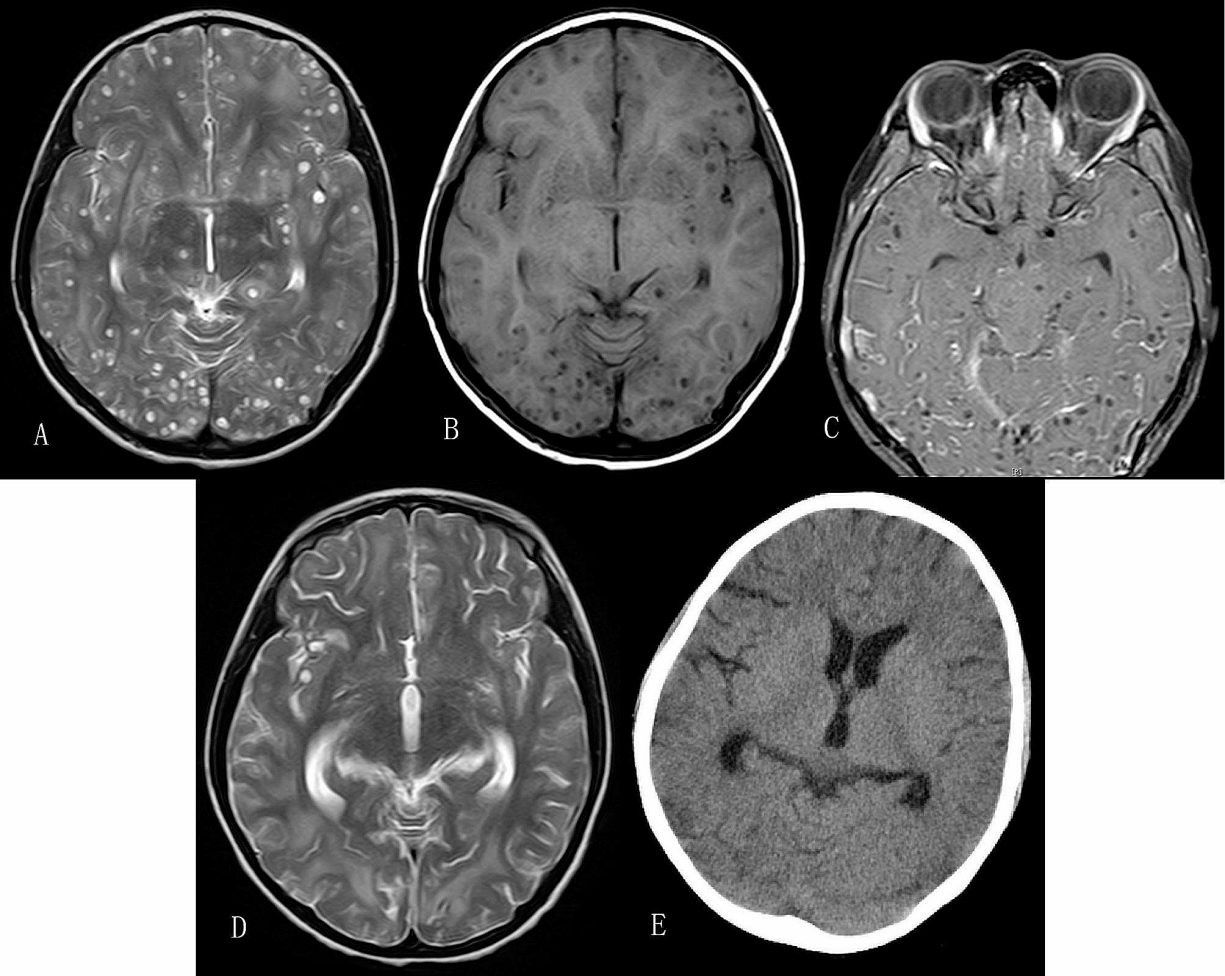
MRI findings of intracranial lesions at baseline and follow-up assessments. Axial T2-weighted (A) and T1-weighted (B) magnetic resonance images before antiparasitic treatment showed massive cystic lesions with the so-called “hole-with-dot” appearance, indicating viable cysticercus with eccentric scolex, which has been regarded as pathognomonic characteristic of neurocysticercosis. C: Contrast-enhanced MRI image of the eyes of the patient which also showed some intracranial lesions before antiparasitic treatment. D: Brain axial T2-weighted MRI image two months after antiparasitic treatment demonstrated resolution of most intracranial cystic lesions. E: Brain CT scan six months after antiparasitic therapy showed very few residual calcifications
